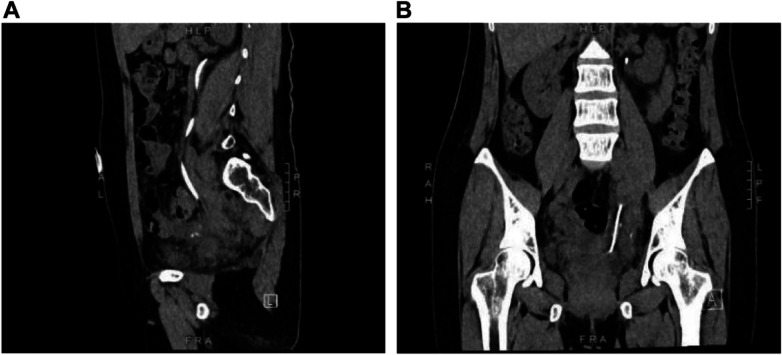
Postoperative abdominal non-contrast computed tomography (August 27, 2025). (A) Abdominal CT sagittal reconstruction section; (B) Abdominal CT coronal reconstruction slice.
